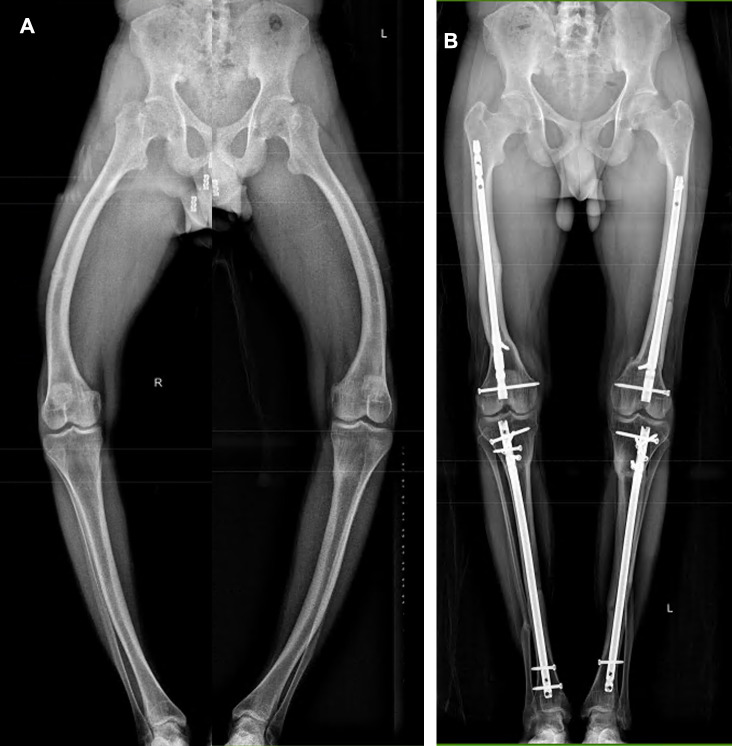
a case of varus alignment with greater femoral varus; A: pre-op standing full-length radiographs, B: full-lengthen radiographs at final fallow-up
